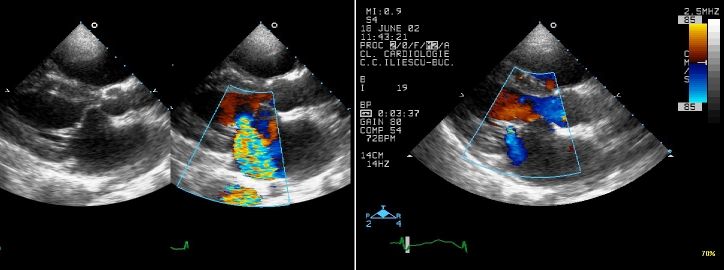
Two D (2D) Transthoracic echocardiography:
